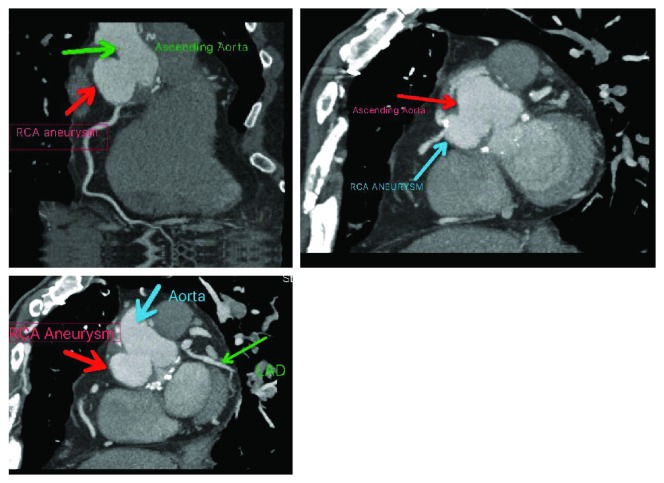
CT scan showing the right coronary artery pseudoaneurysm measuring 2.5 × 4 × 4 cm arising off the right lateral aspect of the aorta. Also shown is the sinus of Valsalva aneurysm measuring 2.4 × 3.8 × 4.0 cm in size (marked “Ascending aorta” and “Aorta”).
